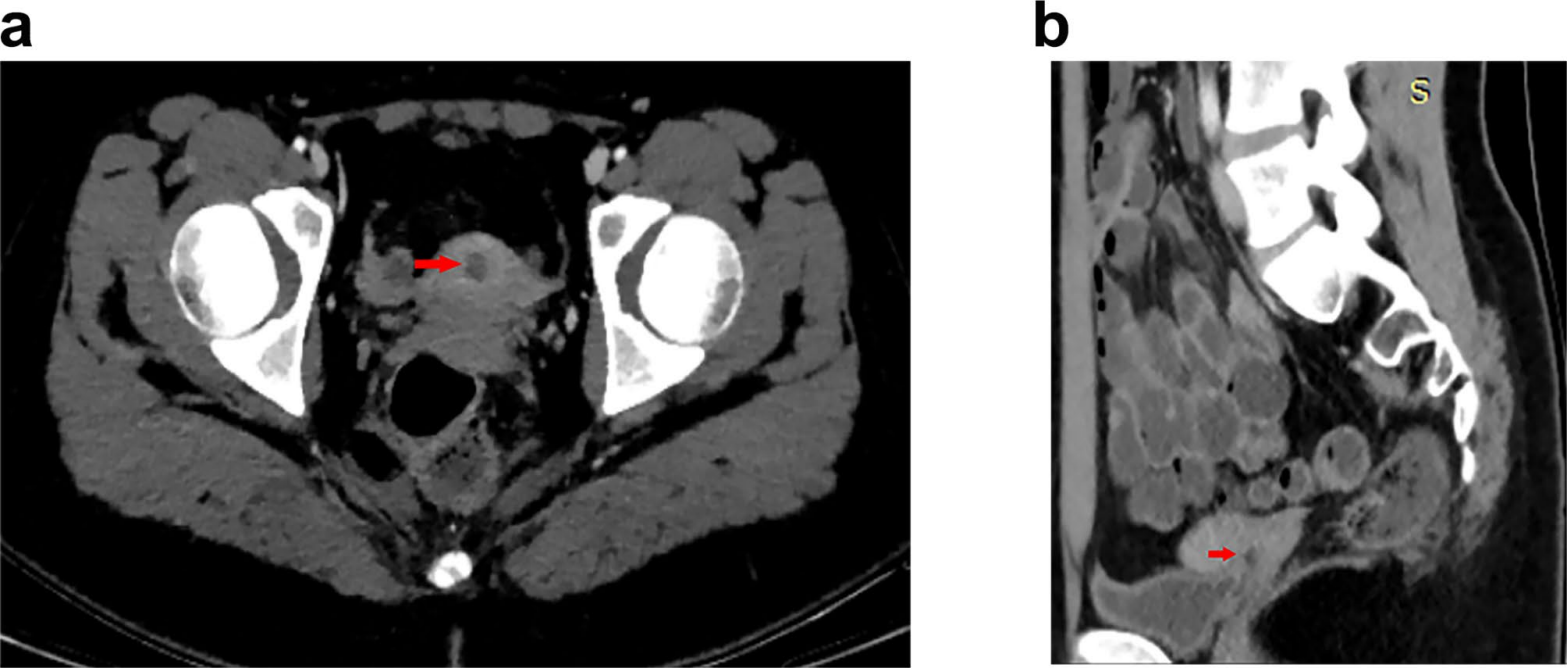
Pelvic CT: a lesion about 1 cm in the douglas sac
